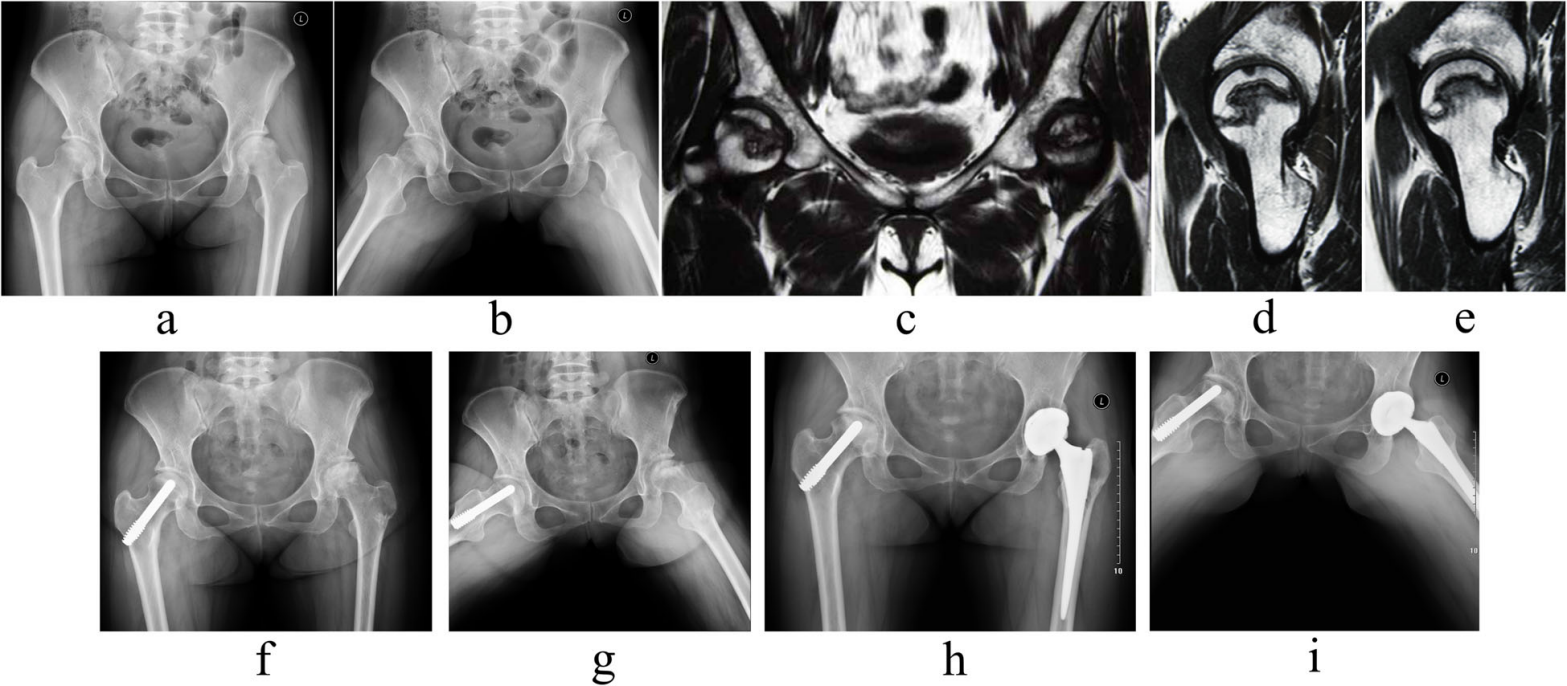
a, b Anteroposterior and frog lateral X-rays of bilateral hips showed that right hip was in ARCO stage II and JIC type C1. c, d, e preoperative MRI images showed right hip was involved serious ONFH but the lateral column was not involved. f, g the immediate radiographs followed by PTRS of right hip were shown. h, i even though mild subchondral bone collapse was observed, intact joint space was achieved with matched femoral head morphology at 9 years postoperatively, and the right hip was successfully preserved
